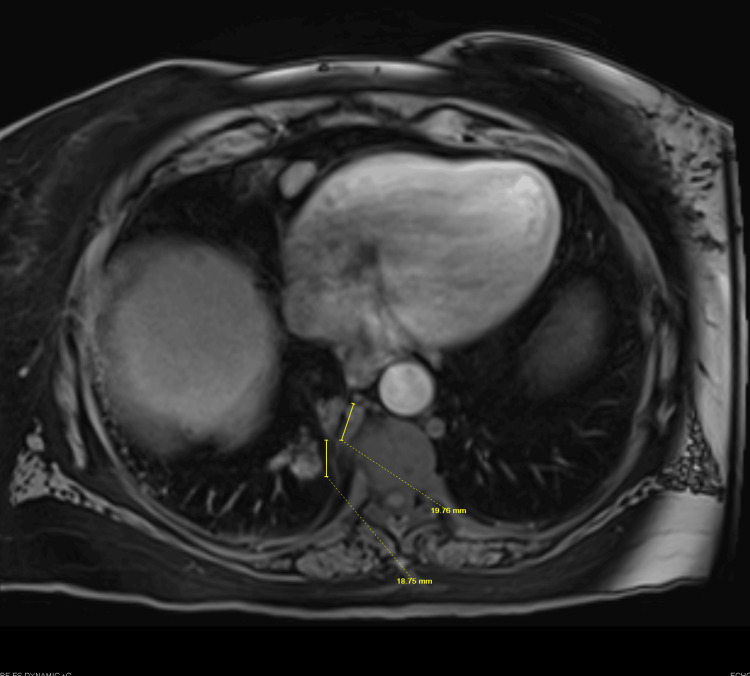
MRI showing incidental nodular lesions yellow arrows in the right lower lobe of the lung MRI: magnetic resonance imaging
